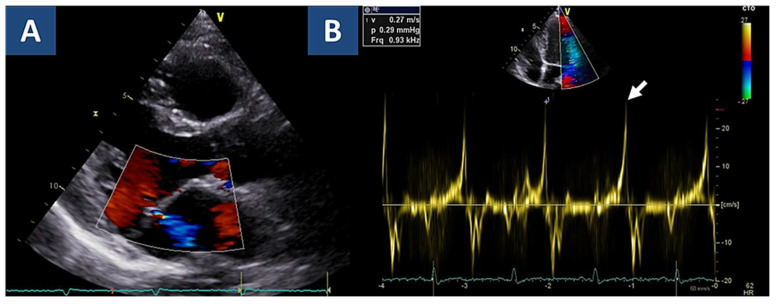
Echocardiographic findings. Legend. (A) Parasternal long axis view shows mild mitral valve regurgitation. Associated curling of the basal inferolateral wall is shown as Supplementary Video. (B) As a hallmark of increased arrhythmic risk, Pickelhaube sign (spiked systolic lateral mitral annular velocity >16 cm/s) is shown (arrow).
